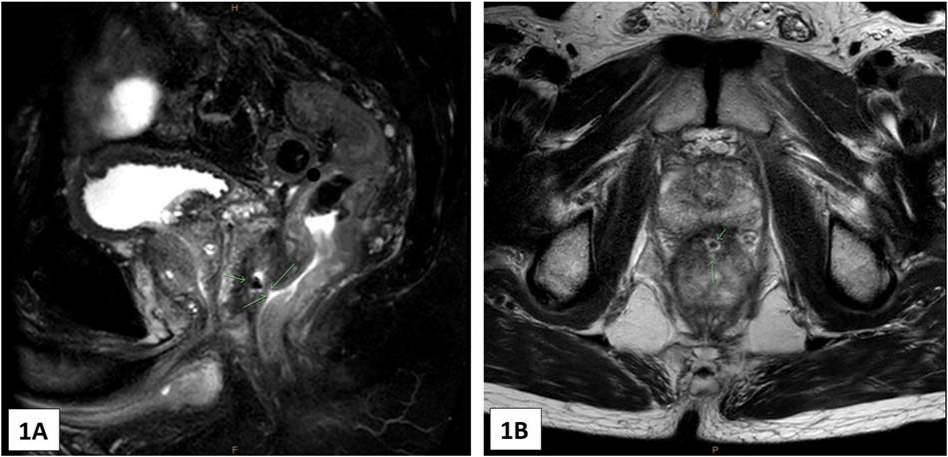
(A) and (B)—MRI images (A in sagittal, B in axial) showing the 3 mm tract from the low rectum extending into the recto-prostatic angle. This is in connection with a small collection at the recto-prostatic angle.
